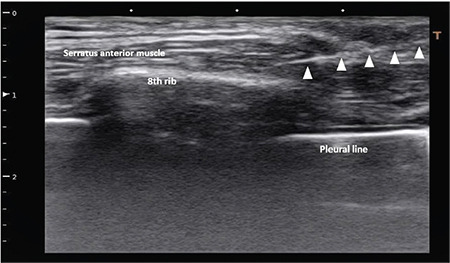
Ultrasound view for the SIPB block, showing the 8^th^ rib with the covering Serratus muscle layer, and the tip of the catheter floating in the local anaesthesia bolus. SIPB, serratus-intercostal plane block.
